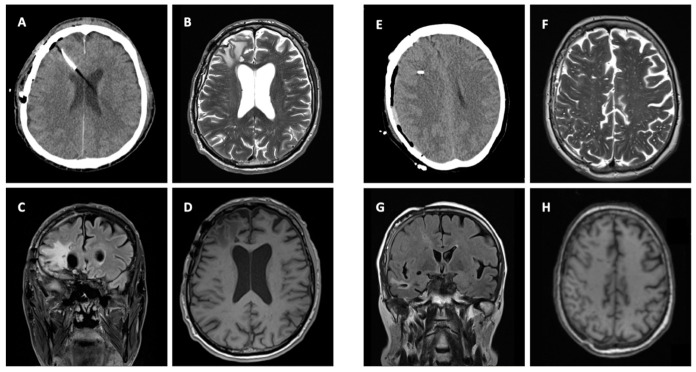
Two exemplary MRI lesions after removal of ICP probe. Panel (A,E) show a cerebral CT scan with probes in place. Panel (B,F) show axial T2-weighted MRI after removal of the probe. Panel (C,D,G,H) show corresponding Flair MRI after probe removal.
